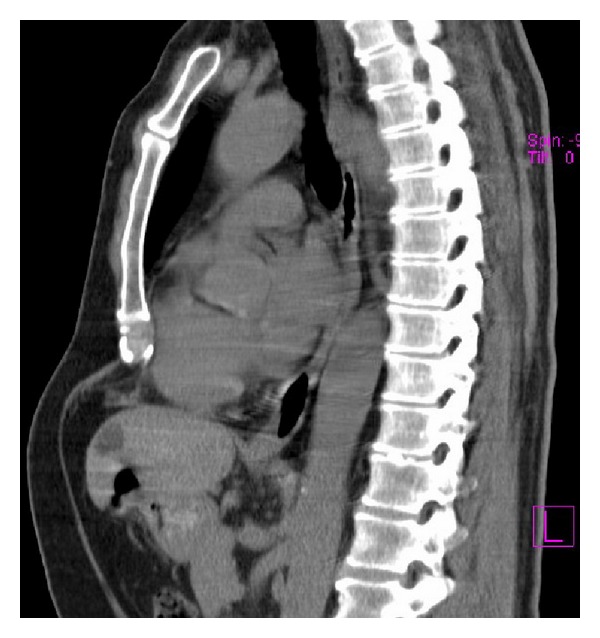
CT chest image of a male patient with PE.
